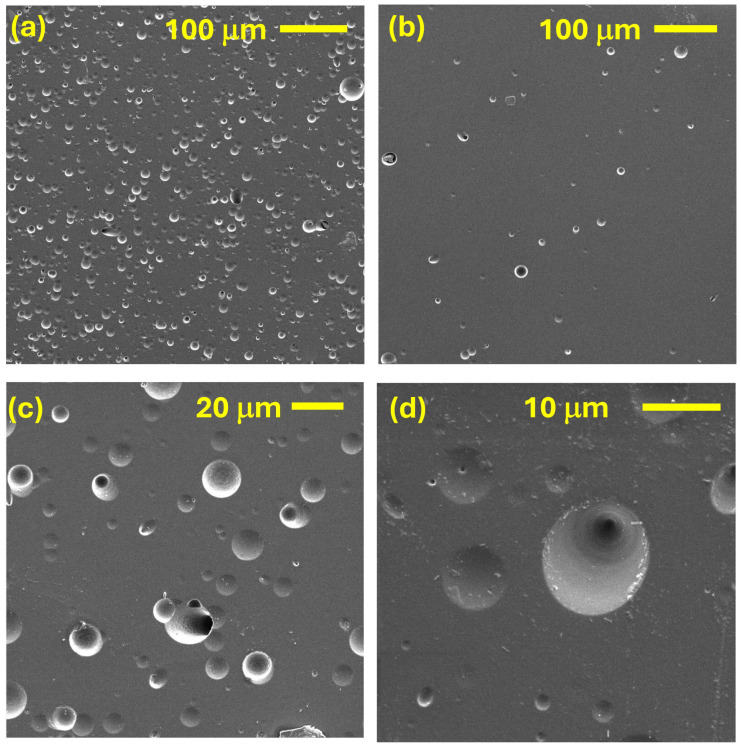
SEM images of an irradiated CR-39 dosimeter on VESUVIO in a region near the beam center (a) and in a region away from the beam center (b). Two images at higher magnification of intermediate regions are also shown in (c,d).
